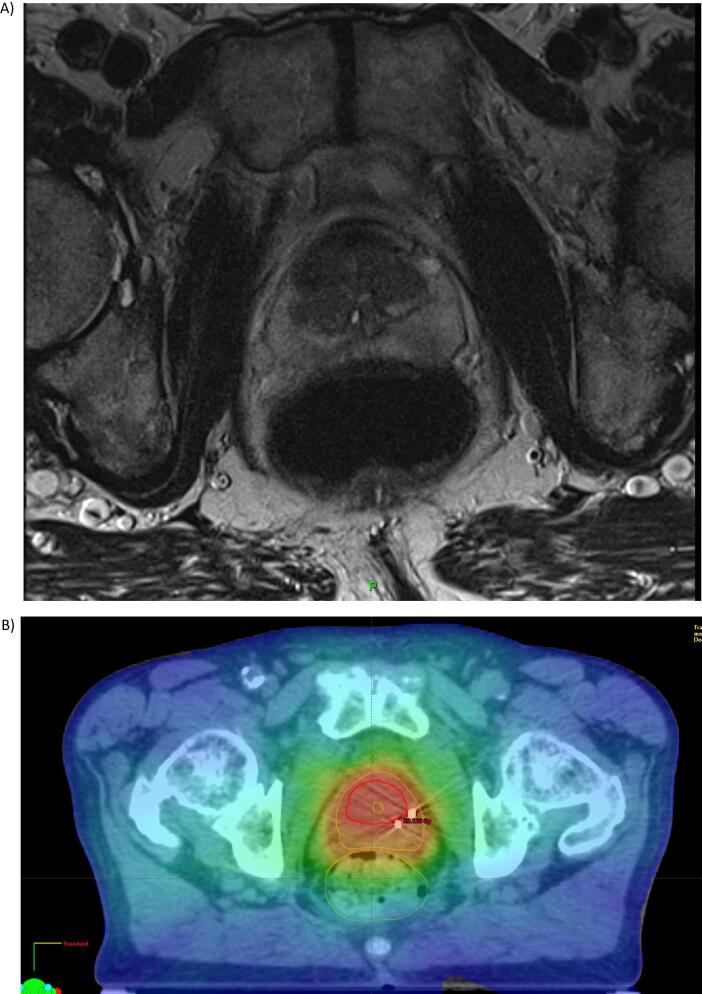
image of a suitable focal prostate boost. A) Multiparametric MRI scan at the mid gland level showing a large tumour in the central zone. B) Corresponding Planning CT scan with dose distribution around the boost volume (red contour) dose level 67 Gy (red colour)), prostate volume (orange line) dose level 60 Gy (orange colour).
